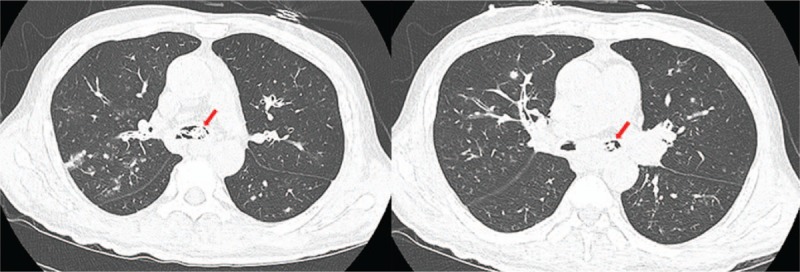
Chest radiography after cardiac arrest. Chest computed tomography showing massive materials in the trachea and both bronchi and no interval changes in centrilobular nodules with confluent consolidation in both the lungs. Arrow: endobronchial materials in both the bronchial trees.
